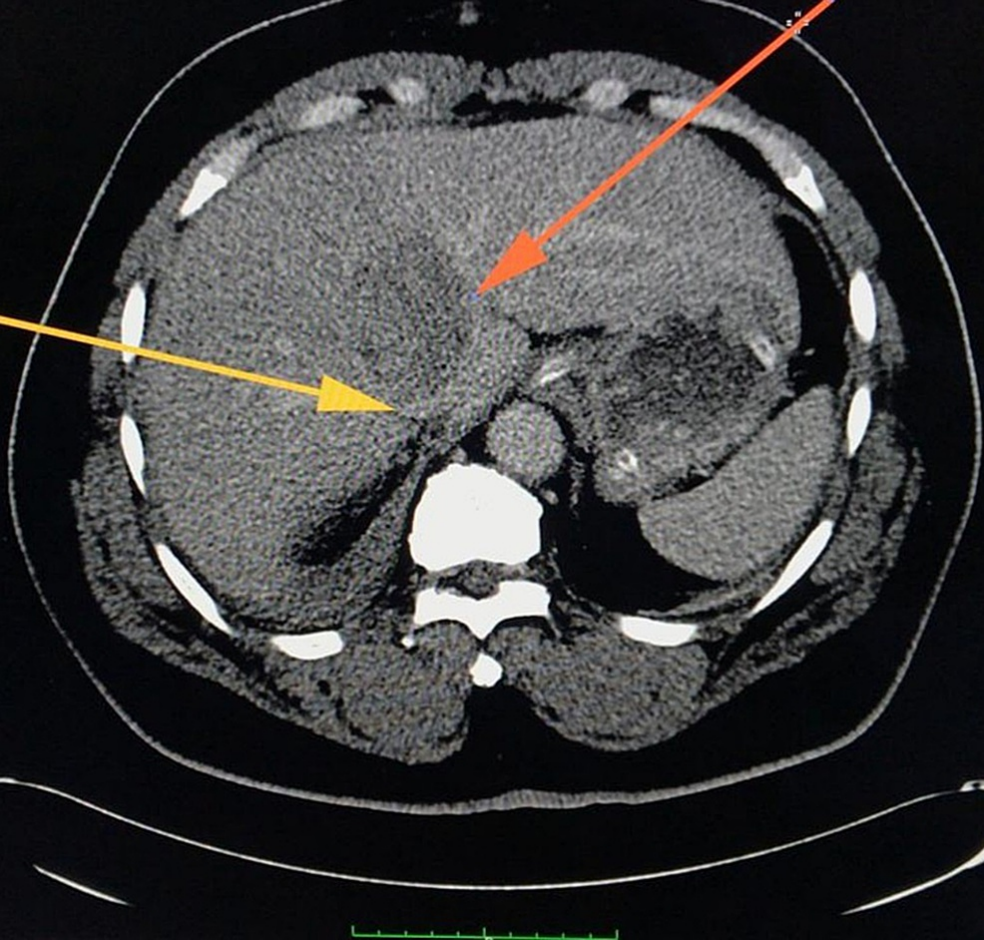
CT scan of abdomen and pelvis with intravenous contrast showing haematoma (orange arrow) compressing the inferior vena cava (yellow arrow) with no extravasation of blood
